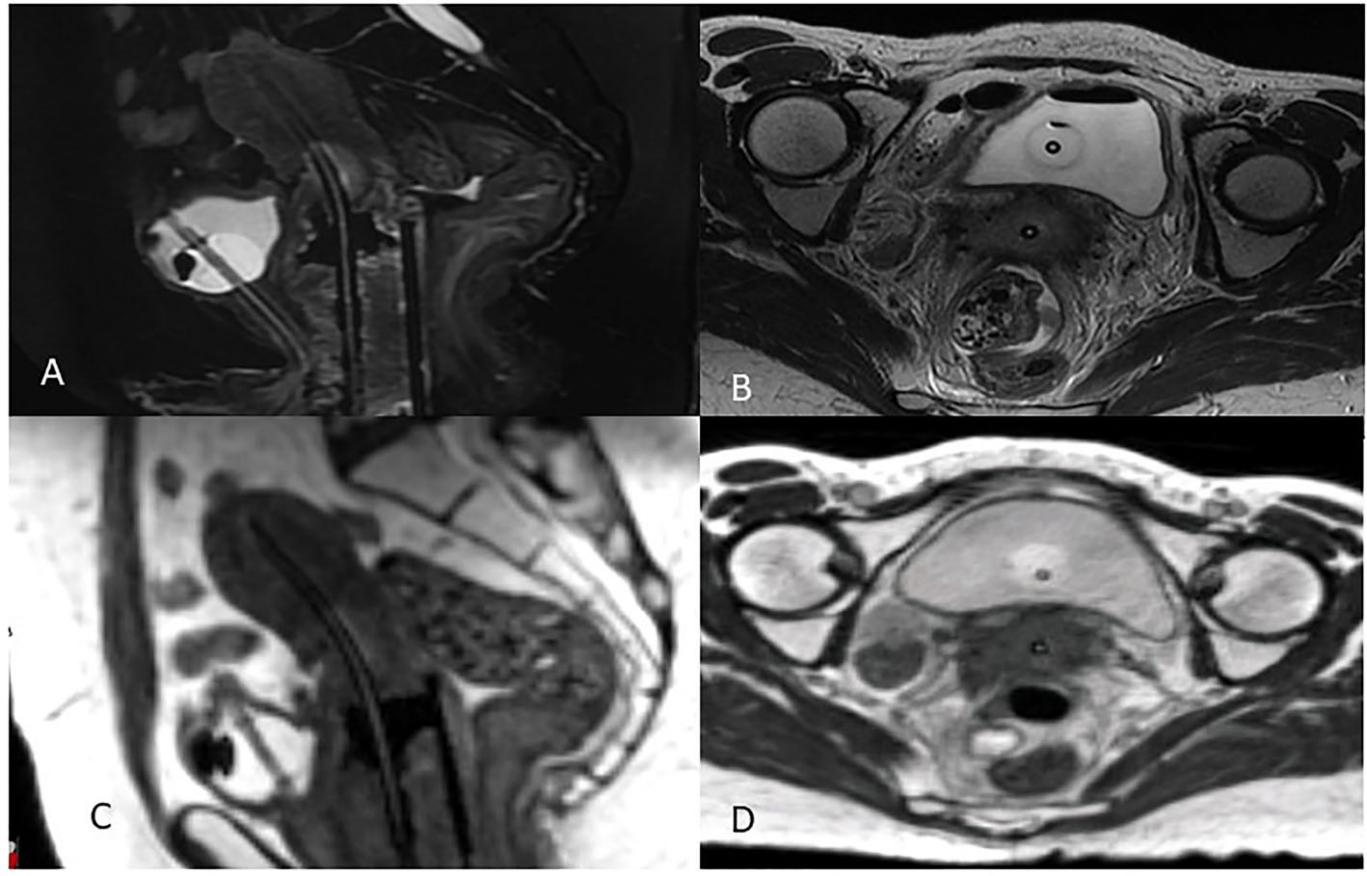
MRg Brachytherapy 3T Diagnostic MRI (A) Sagittal and (B) axial views compared to 0.35T MRIdian MRI (C) Sagittal (D) axial views.
